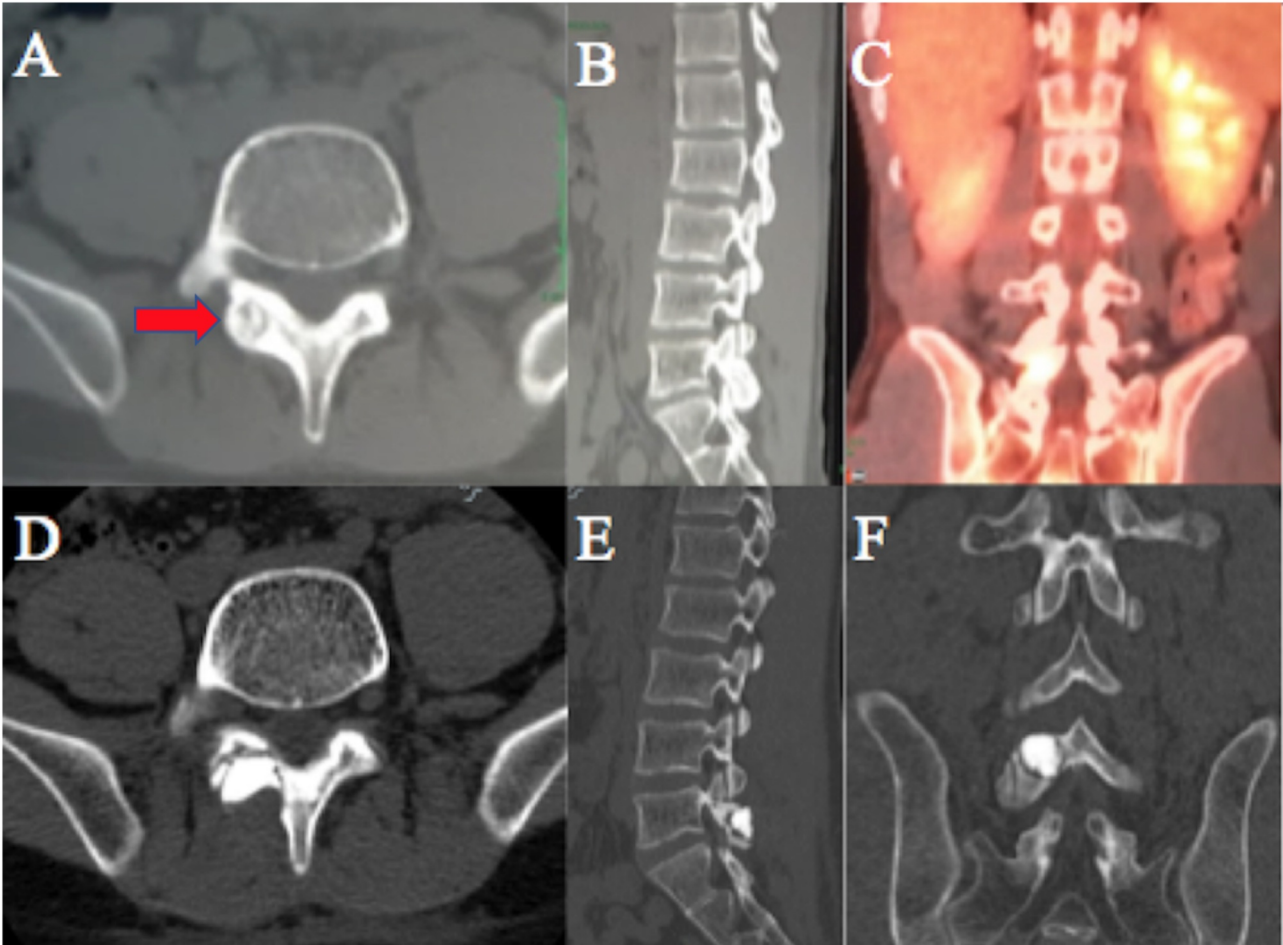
Pre-operative computed tomography (CT) imaging (A) pre-op axial CT showing an 11 x 10 mm sclerotic lesion in the right L5 lamina (red arrow); (B) right L5-S1 foraminal narrowing due to the laminar lesion; (C) positron emission tomography-computed tomography (PET-CT) imaging showing a metabolically active right L5 lamina; (D,E,F) postop axial, sagittal, and coronal CT scans, respectively, after resection of osteoid osteoma with cement augmentation.
